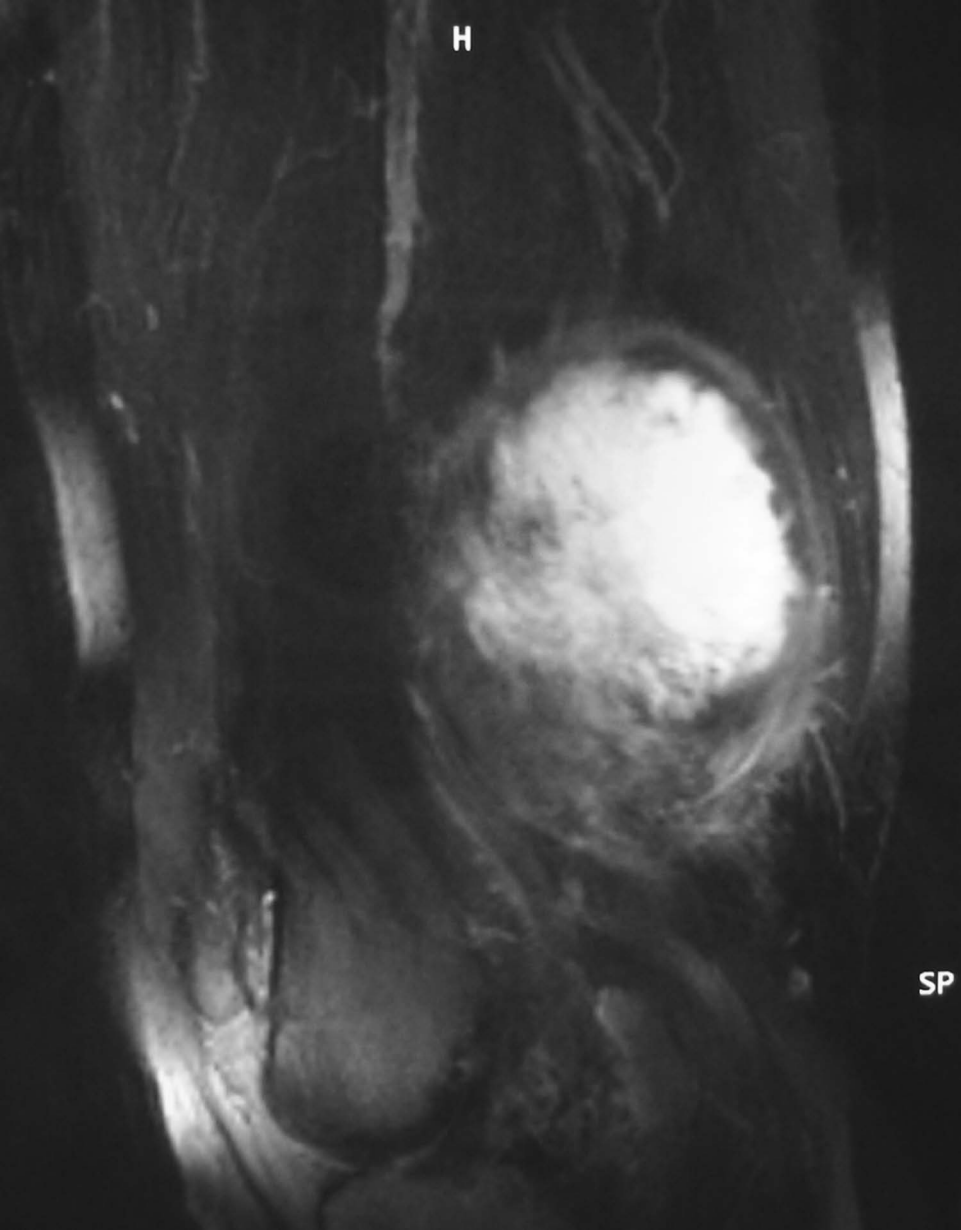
"T1-weighted, fat-suppressed, contrast-enhanced on a sagittal plane MR image revealed a hyperintense central part and a hypointense irregular wall corresponding to myxoid and fibrous pattern, respectively."
